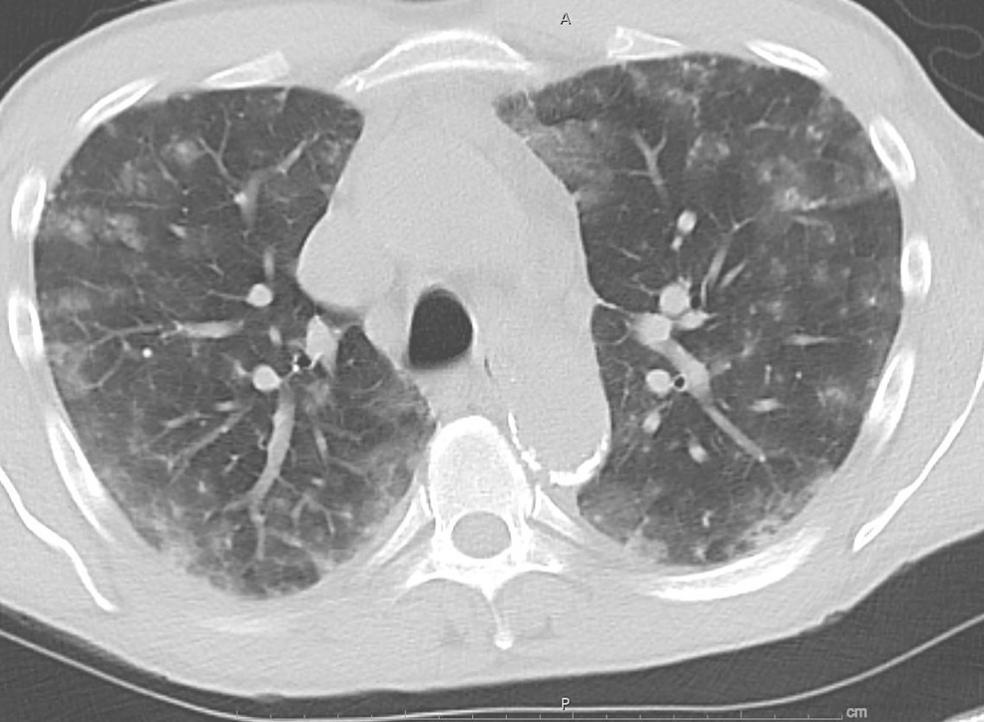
CT scan of the chest without contrast showing nonspecific diffuse ill-defined pulmonary nodules and ground-glass opacities CT: computed tomography
